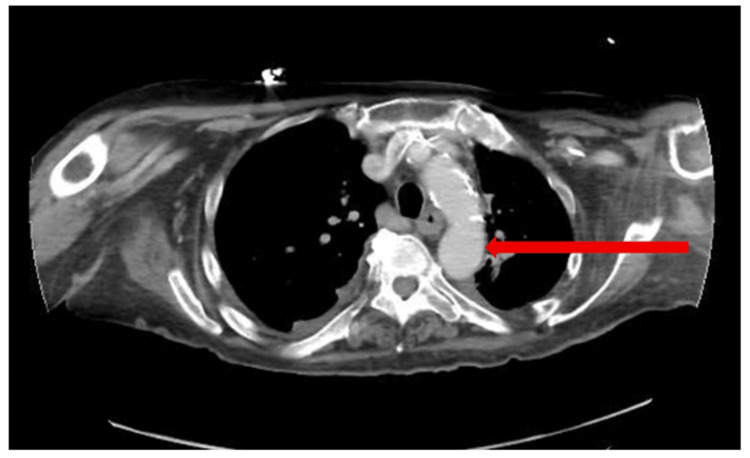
Repeat axial view CTA imaging demonstrating resolution of the descending thoracic aorta mobile thrombus, as demonstrated by the red arrow marking a comparable aortic zone, following completion of six months of aspirin monotherapy CTA, computed tomography angiography
